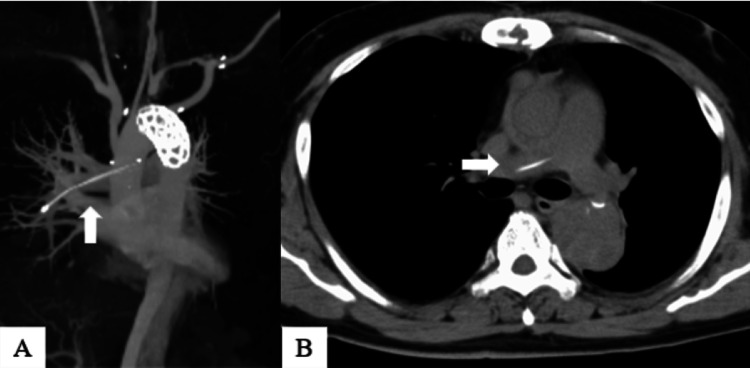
Computed tomography scan before additional thoracic endovascular aortic repair. (A) White arrow: residual TEPW migration to the right pulmonary artery. (B) Another view in the same computed tomography as (A) (white arrow: residual TEPW in the right pulmonary artery). TEPW: Temporary epicardial pacing wire
